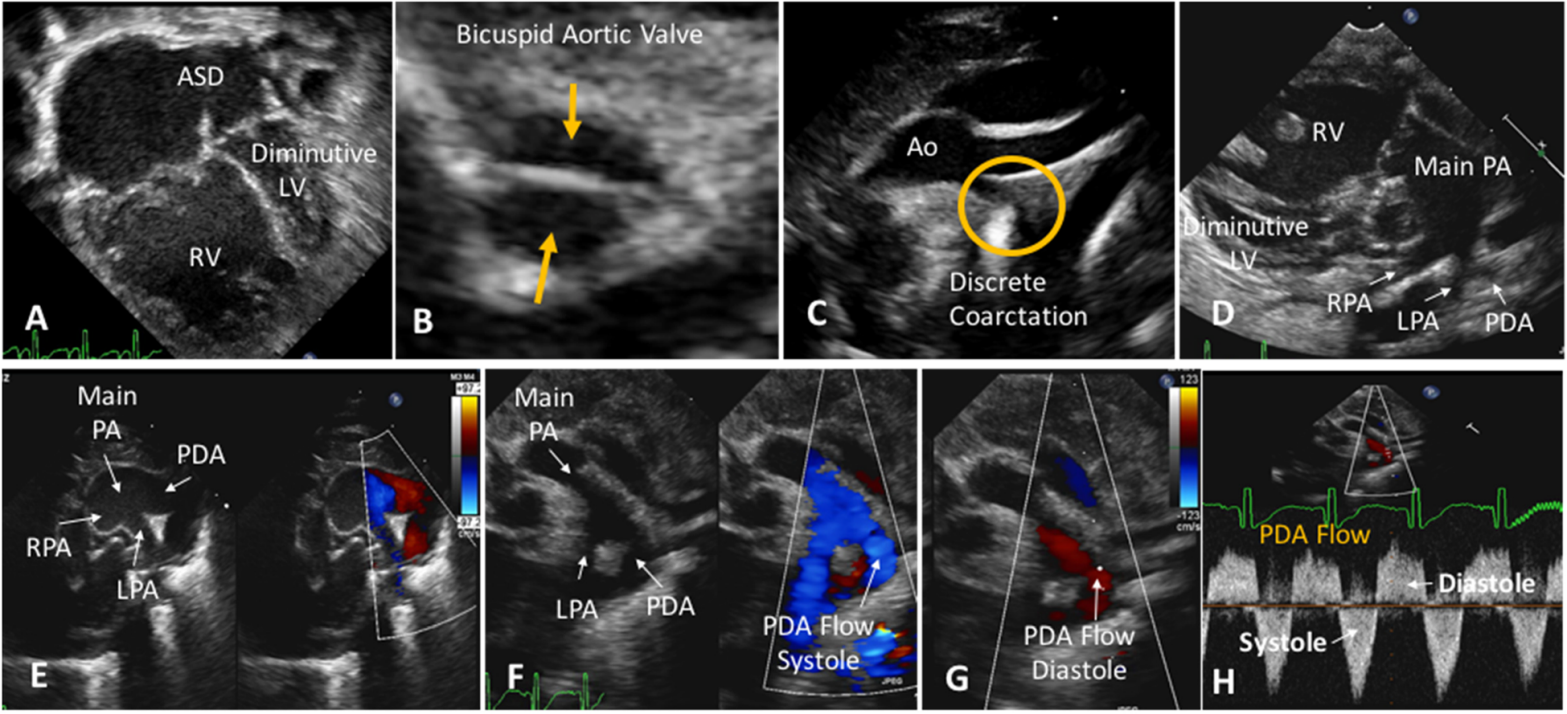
Echocardiogram findings for IV.3. Legend: a Large atrial septal defect (ASD) with a diminutive/hypoplastic left ventricle (LV); RV-right ventricle. b Orange arrows point to bicuspid aortic valve leaflets. c Hypoplastic aorta (Ao) with a discrete coarctation. d and e Two views of the patent ductus arteriosus (PDA); PA -pulmonary artery; RPA - right pulmonary artery; LPA - left pulmonary artery. f-g-h Color and spectral Doppler of the to-and-fro PDA flow from the PA and Ao
